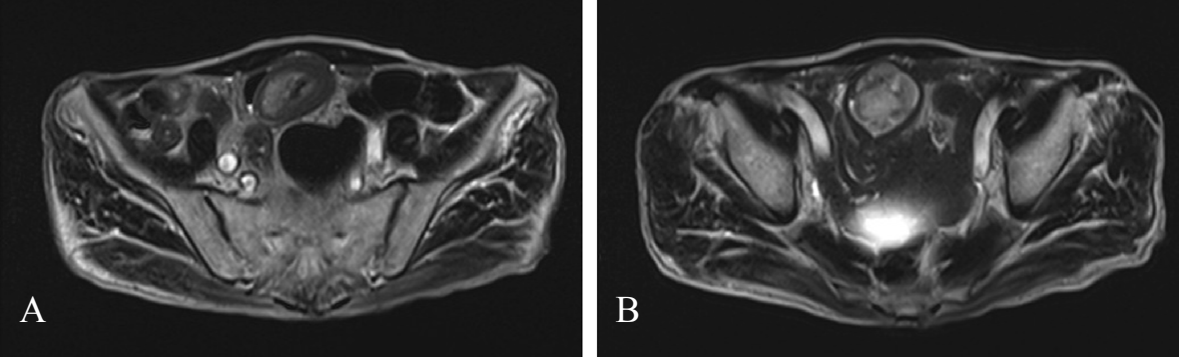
T2-weighted magnetic resonance image. Reveals an intussusception as a target-like mass (A) and a hyper-intense tumor as the lead point (B).
